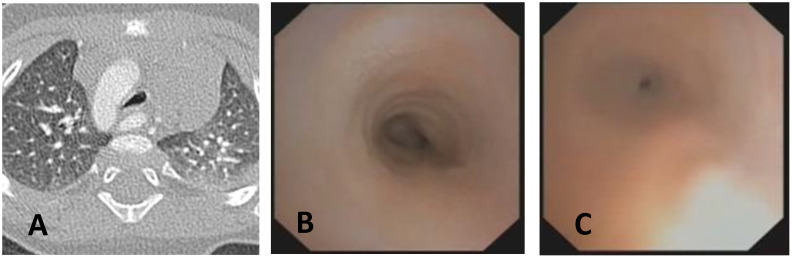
(A) Chest CT with CM: right aortic arch with lusory subclavian artery and marked deformation of tracheal lumen at this level. (B) SVBS: a patent tracheal lumen is present up to the junction between the middle and distal third tracheal lumen, where pulsating extrinsic compression appears on the anterolateral right wall, deforming and narrowing the tracheal lumen. The carina is patent, but the main right bronchus inlet is slightly deformed by the aortic arch. (C) DVBS, stimulating cough reflex: Almost complete occlusion of trachea. Abbreviations. CT: Computed Tomography; CM: Contrast Medium; SVBS: Static Videobronchoscopy; DVBS: Dynamic Videobronchoscopy.
